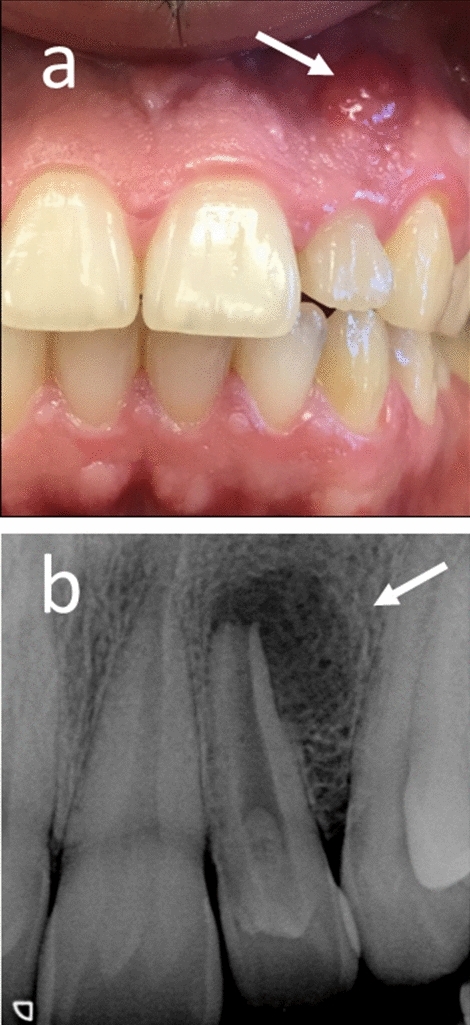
Complication: failure to diagnose dens invaginatus in time. Failure to diagnose in time the dens invaginatus and failure to apply early preventive measures led to pulp involvement, infection and necrosis, resulting in formation a periapical lesion. (a) White arrow indicates a suppurating sinus tract (b) White arrow: periapical bone resorption in response to infection in the root canal.
